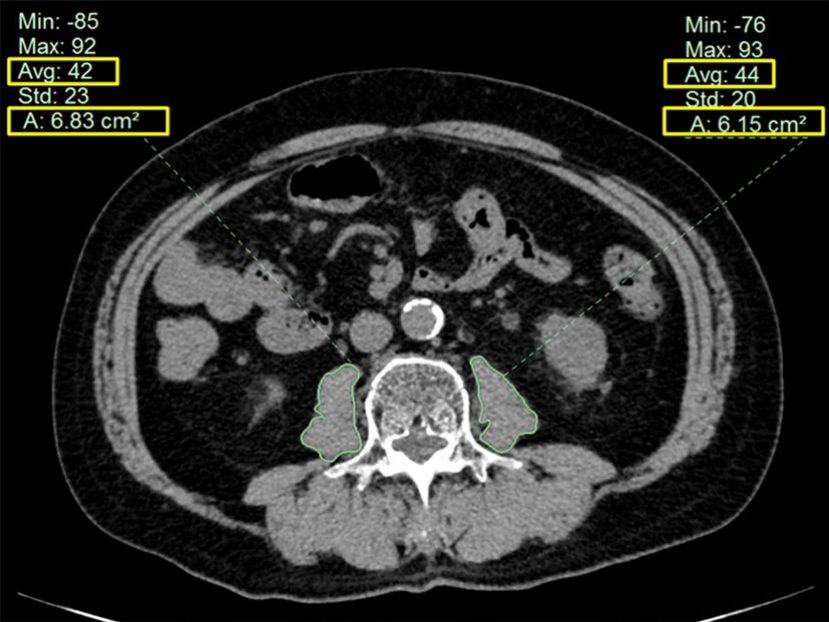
Measurement of area and density of each psoas muscle on the axial non-contrast computed tomography image at level L3.
